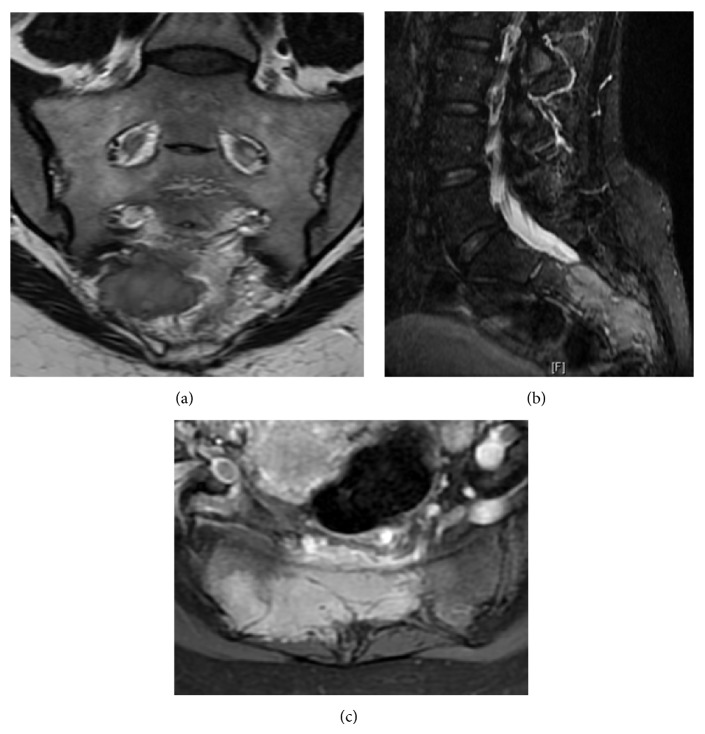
Preoperative T2-weighted contrast enhanced MRI showing an expansive and osteolytic lower and mid-sacral lesion, extending up to the inferior half of S2 vertebra, with both intra- and extracanalar components and a ventral extension displacing the rectum anteriorly. (a) Coronal, (b) sagittal, and (c) axial views.
